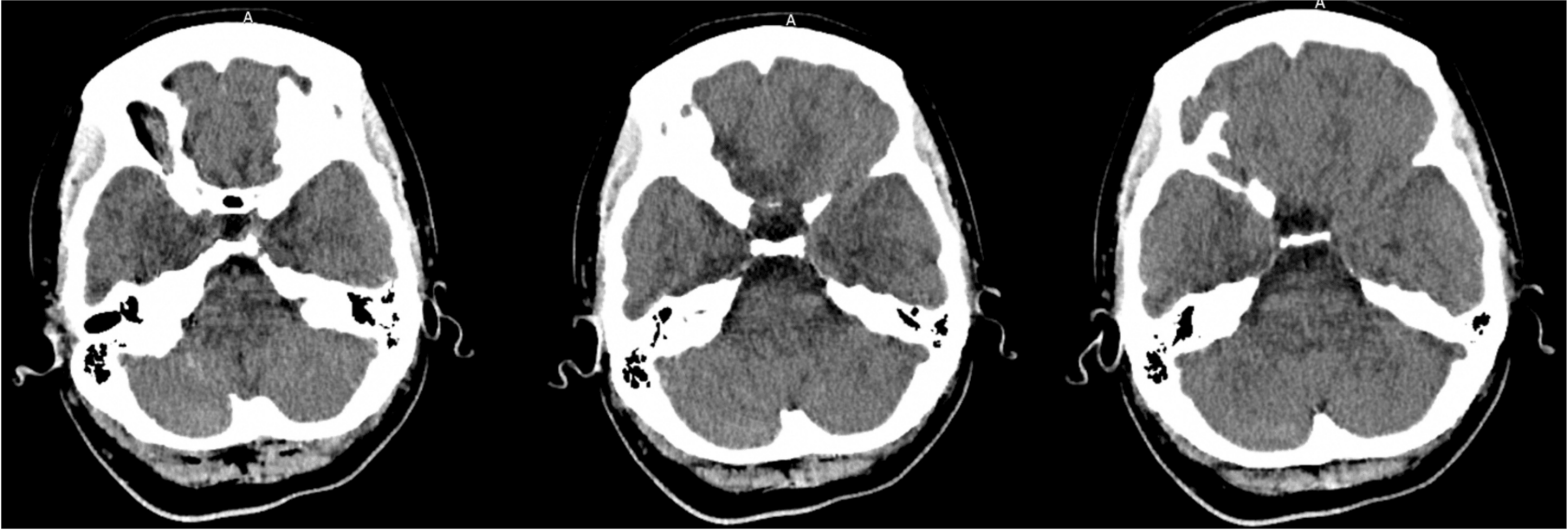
Non-contrast CT of the head. Effacement of the basal cisterns and crowding of the foramen magnum, which is likely secondary to diffuse cerebral edema. There was no significant opacification of intracranial arteries. There was opacification of external carotid artery branches.
